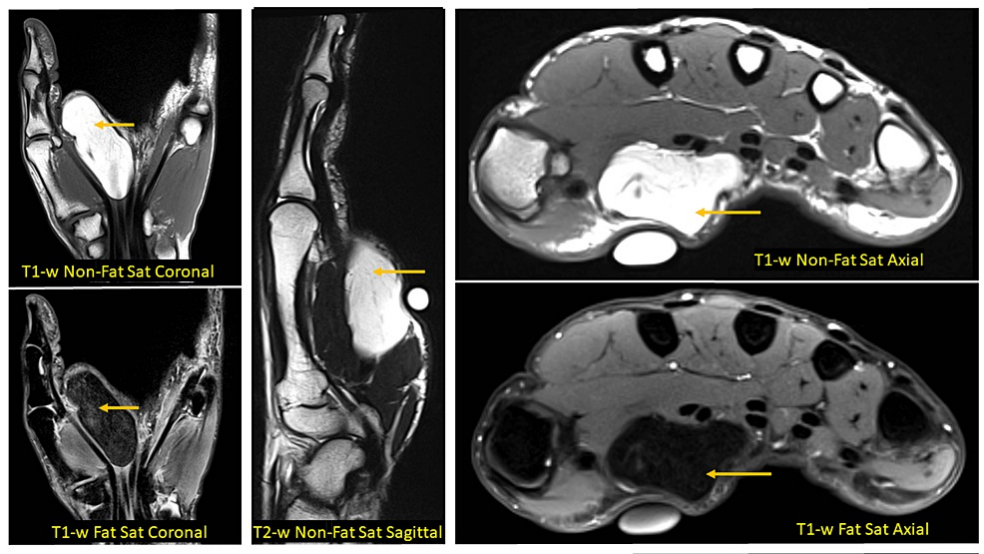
Magnetic resonance imaging findings: coronal, sagittal, and axial sections of the hand showing the mass among the thenar muscles.
